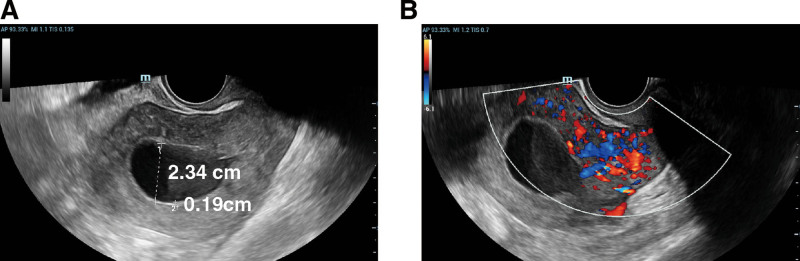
The preoperative transvaginal ultrasound examination of the uterus and bilateral adnexa. (A) Separation of the uterine cavity line; (B) increased echogenicity in the middle-lower segment of the uterine muscle layer, with abundant internal vascularity.
